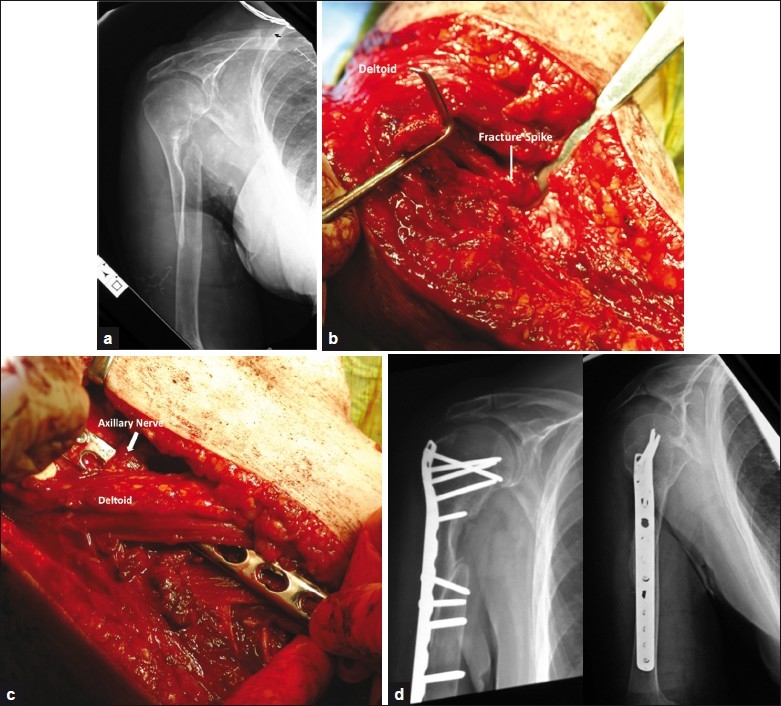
(a) Preoperative radiographs of the patient in case 3; (b) Intraoperative image depicting the lateral surgical approach, with the fracture site identified, prior to debridement; (c) Intraoperative photograph of tunneling the plate under the axillary nerve and the deltoid insertion; (d) Postoperative radiographs
